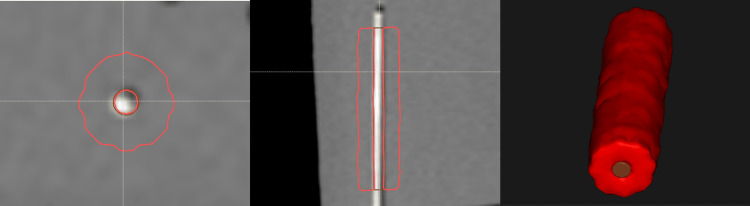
Contours used in analysis of CT images. For each channel, the needle was contoured in addition to a rind around the needle with a wall thickness of 5 mm. The rind region was used to quantify the metal artifact, while both volumes were used to calculate contrast-to-noise ratios.
